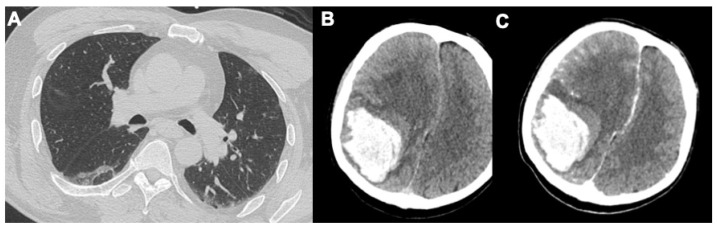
A case of spontaneous hemorrhage in a 56-year-old male. Lung involvement (A) is limited, but there is an extensive right parietal hemorrhage with an evident shift to the left, clear visible in baseline CT (B) and after contrast media injection (C).
